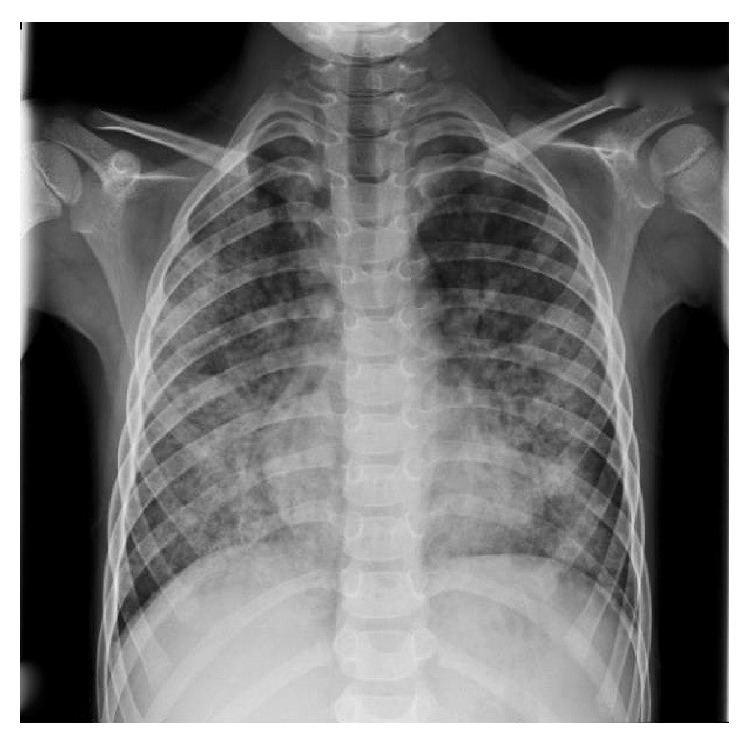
Posteroanterior chest radiography showing diffuse bilateral pulmonary infiltrations.
